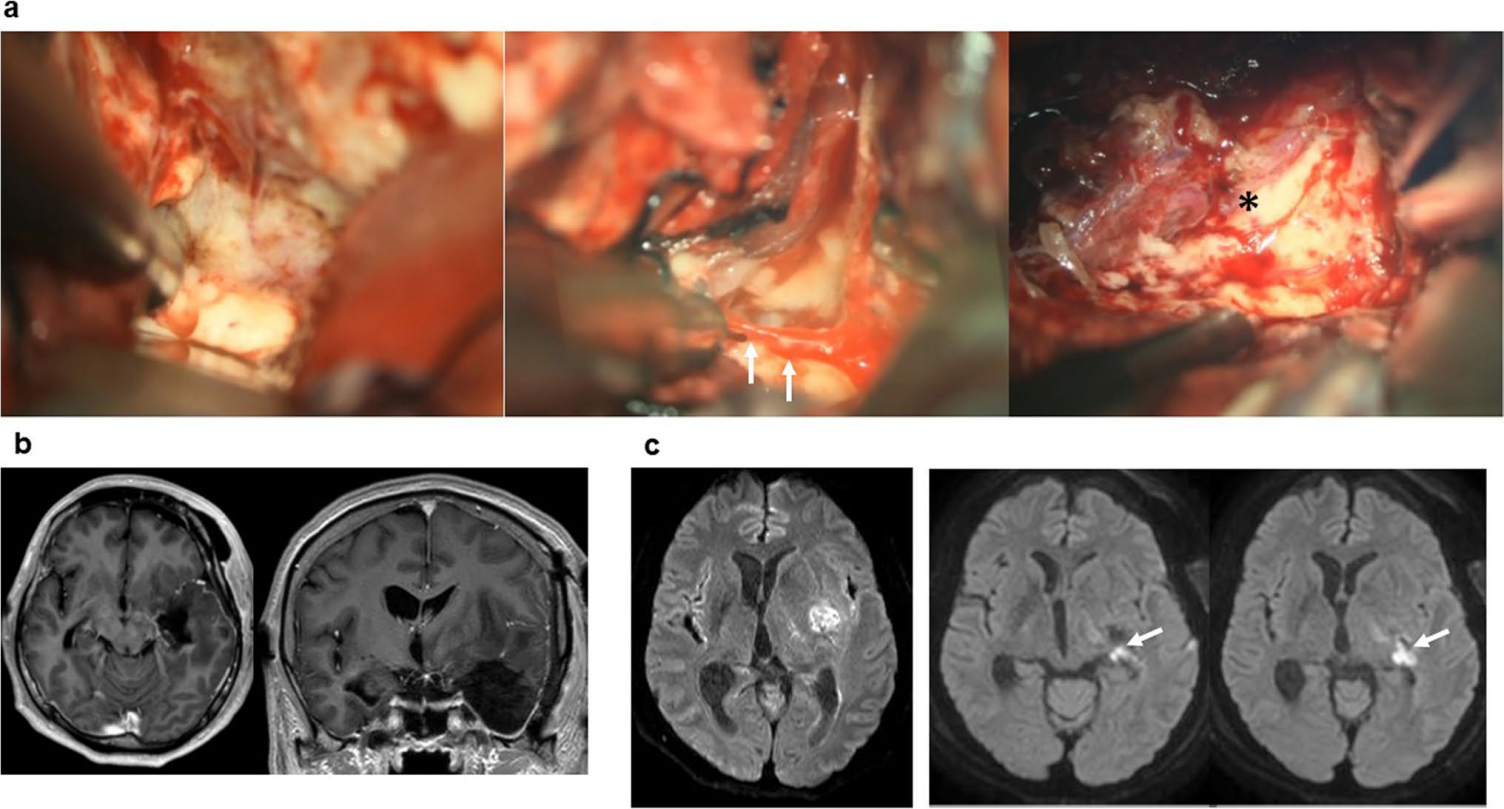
Intraoperative and perioperative magnetic resonance (MR) imaging findings. a Intraoperative views showing the distinct tumor border (left panel), feeding artery from the medial side (arrows, middle panel), and tumor location (right panel). The tumor was located anterocranial to the inferior horn of the left lateral ventricle (asterisk). b Postoperative gadolinium-enhanced T1-weighted MR images showing complete resection of the enhanced lesion. c Pre- (left panel) and postoperative (right panel) diffusion-weighted MR images (DWI) showing a new hyperintense lesion (arrows) in the retrolenticular and sublenticular internal capsule regions compared with preoperative DWI
